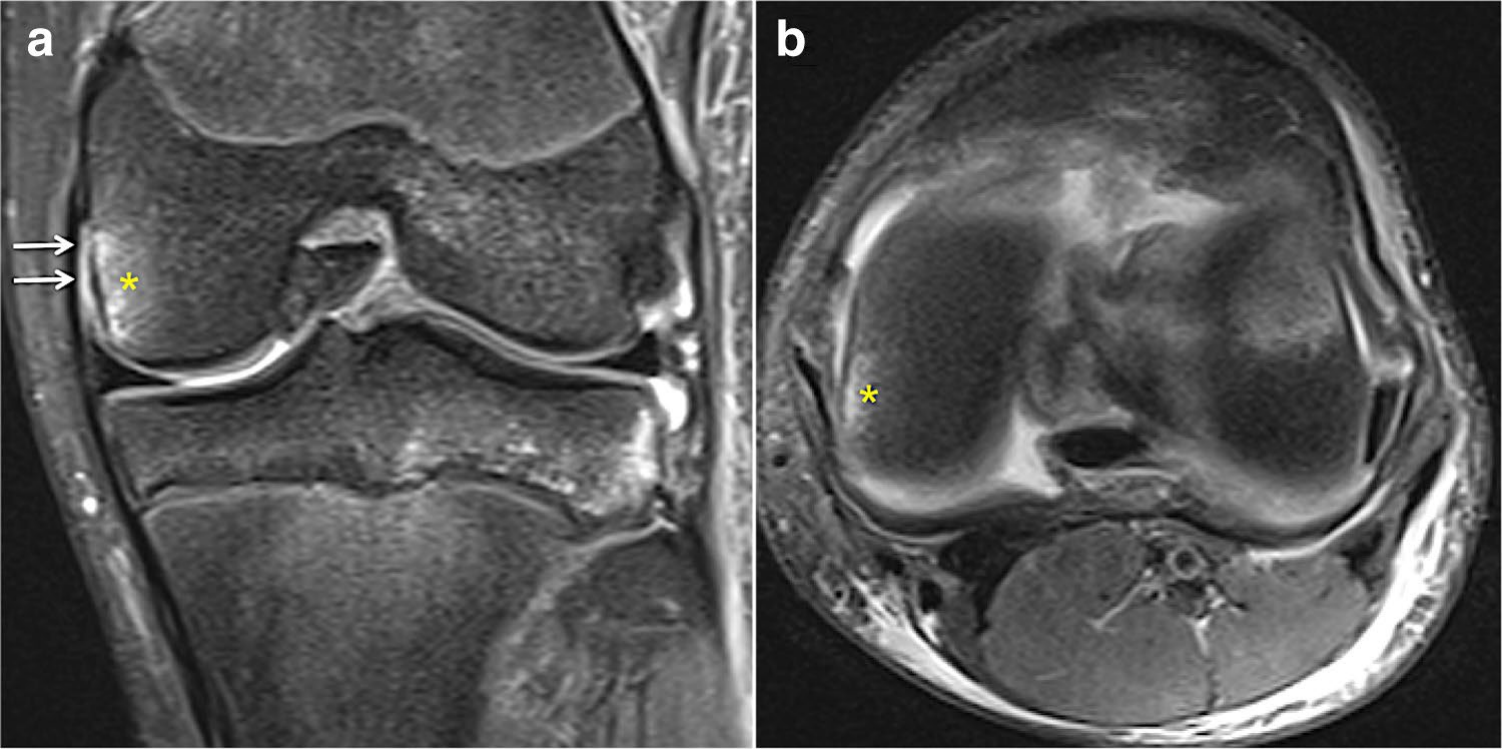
Coronal (a) and axial (b) fat-suppressed proton density-weighted magnetic resonance imaging indicates a dMCL injury (white) with bone oedema (*) at the medial femoral condyle adjacent to the dMCL attachment
